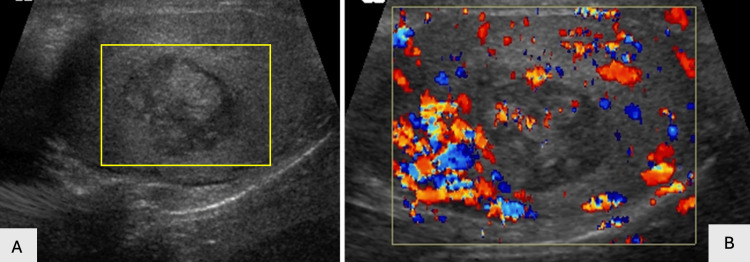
Testicle with a central echogenic abscess (yellow rectangle) on grayscale US imaging (20A). Increased vascular flow surrounding the central testicular abscess seen on color Doppler US (20B).
